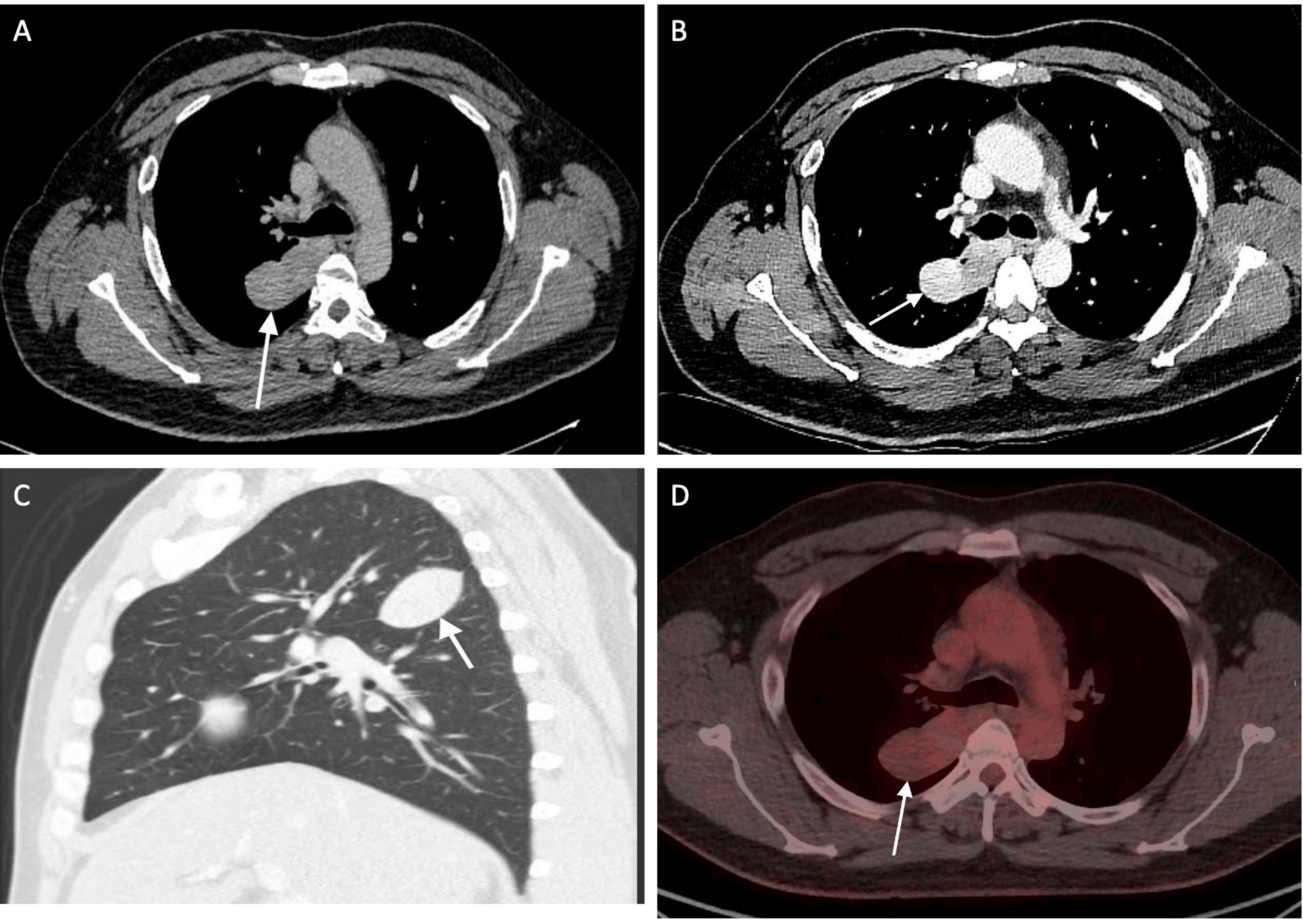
Radiological findings of the Solitary Fibrous Tumour of the Pleura A. Axial section of CT Thorax (non-contrast) showing a bilobed mass. B. Axial section of CT Thorax with contrast showing heterogeneous enhancement. C. Sagittal section of CT (lung window) showing a well-defined mass arising from the right major fissure. D. FDG PET axial window showing right upper lobe mass with low diffuse FDG avidity. FDG PET: Fluorodeoxyglucose positron emission tomography
